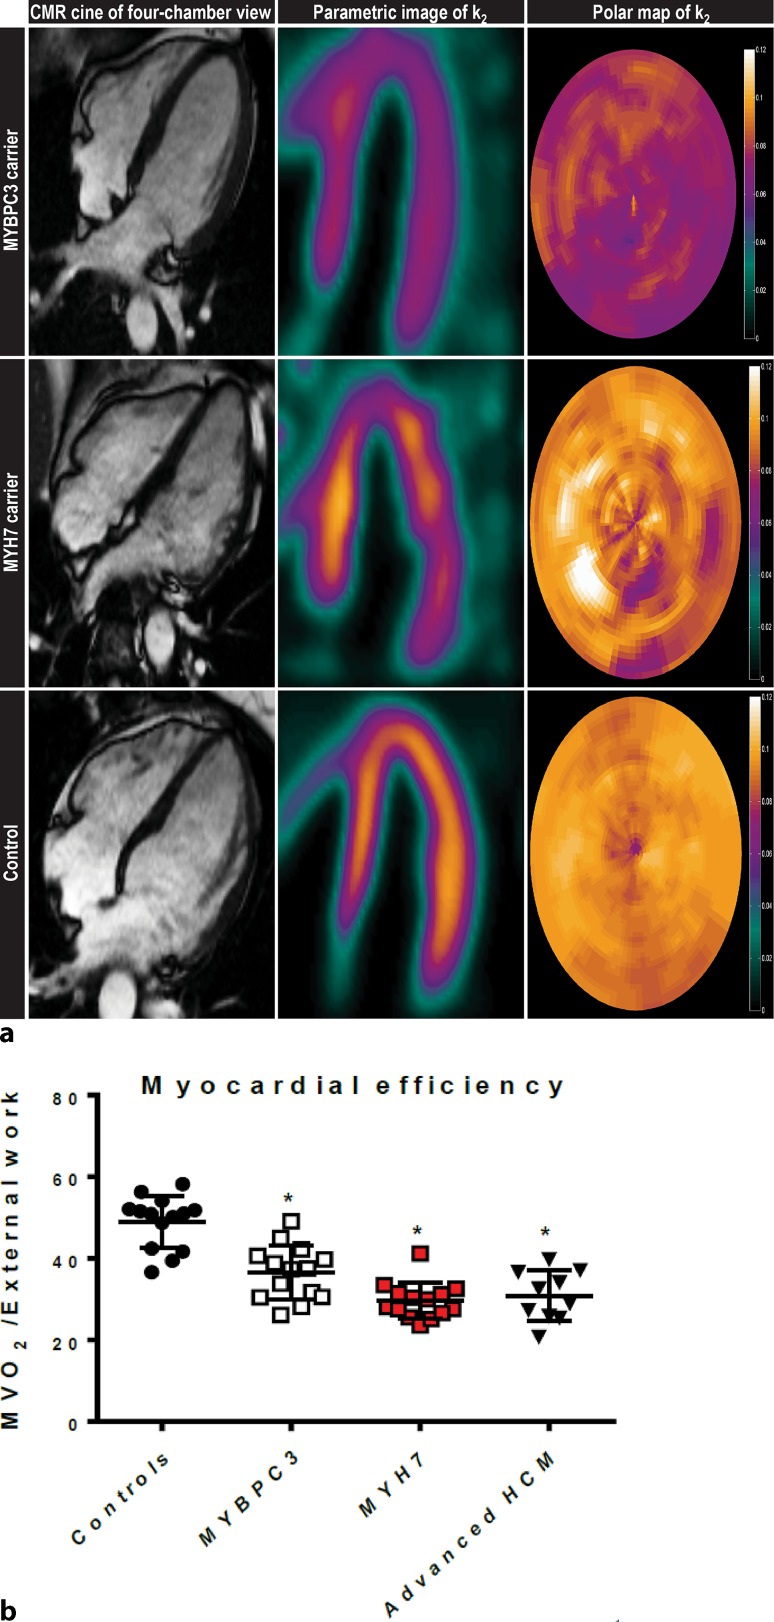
The ratio between external work and myocardial oxygen consumption to obtain myocardial external efficiency was determined in 28 asymptomatic mutation carriers (14 *MYBPC3* and 14 *MYH7*), 10 manifest HCM patients and 14 healthy controls using [^11^C]-acetate positron emission tomography (PET) and cardiovascular magnetic resonance imaging (CMR). a CMR-derived cardiac 4‑chamber view and parametric images of [^11^C]-acetate PET derived k2 with corresponding polar maps. As can be seen clearly, oxygen metabolism was higher in asymptomatic mutation carriers compared to controls [7]. b Myocardial efficiency is not further reduced in advanced HCM patients compared with mutation carriers [8]
